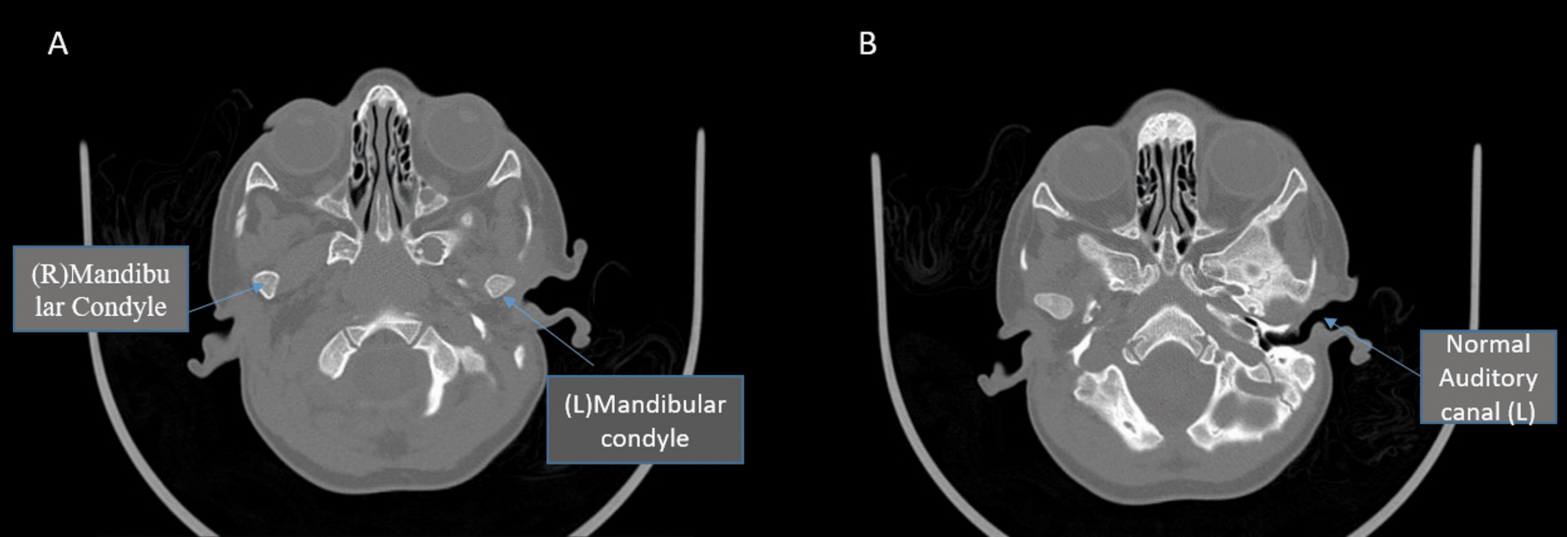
CT scan of facial bones axial view. (A) well formed right and left mandibular condyles and (B) normal auditory meatus over the left side.
